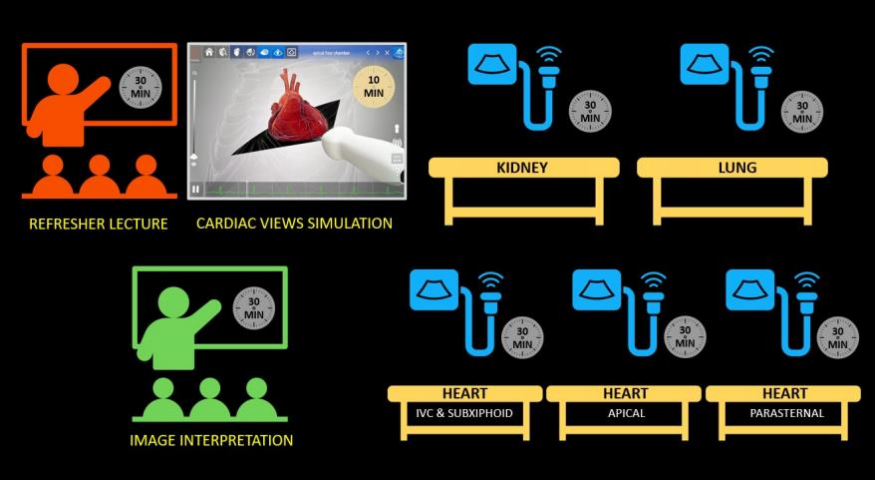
Nephrology Point of Care Ultrasound Workshop Curriculum. Two identical half-day courses were conducted. IVC, inferior vena cava.
